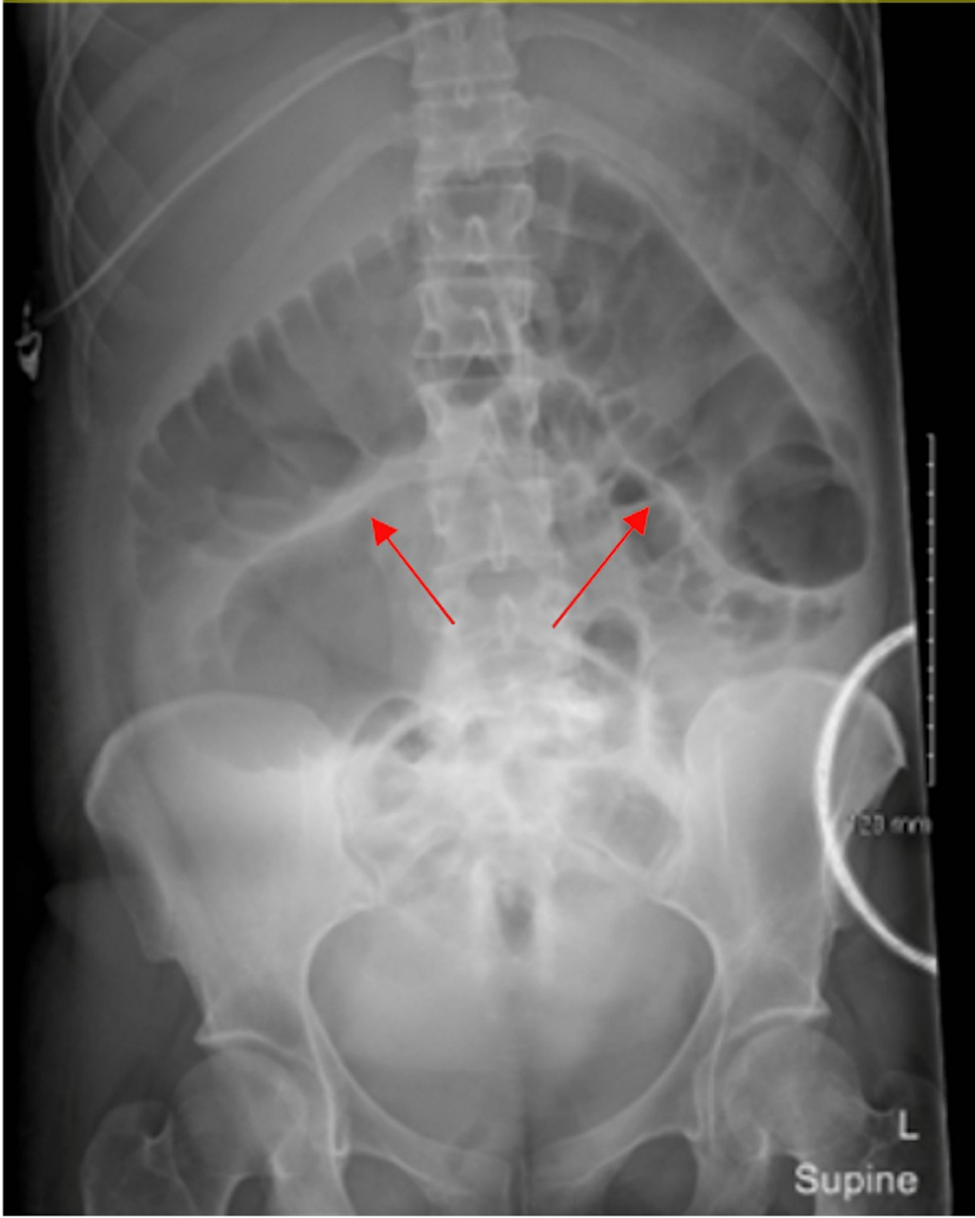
KUB demonstrating dilated loops of the bowel. KUB: X-ray of kidneys, ureters, and bladder
